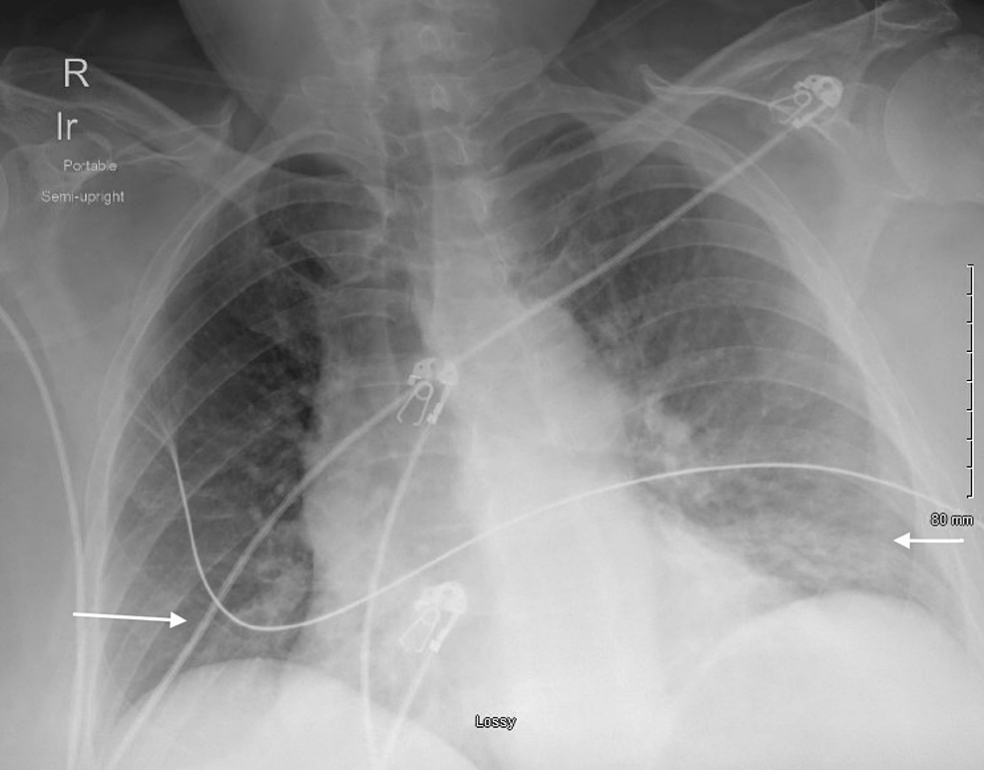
Chest X-ray showing bibasilar infiltrates.
